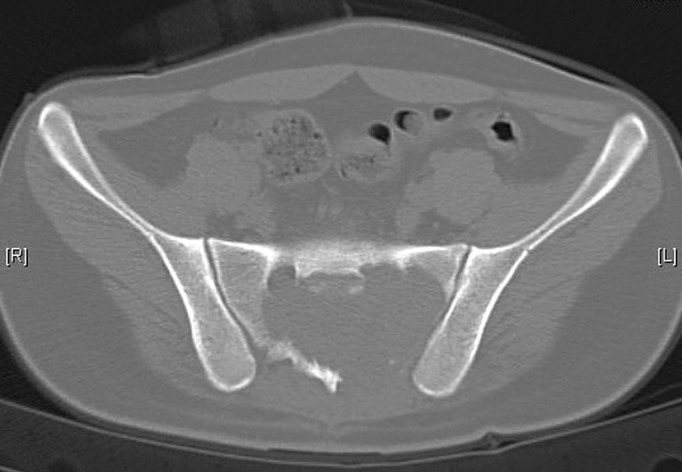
Computer tomography (CT scan) showing a large lytic lesion involving the sacrum (Case #2).
